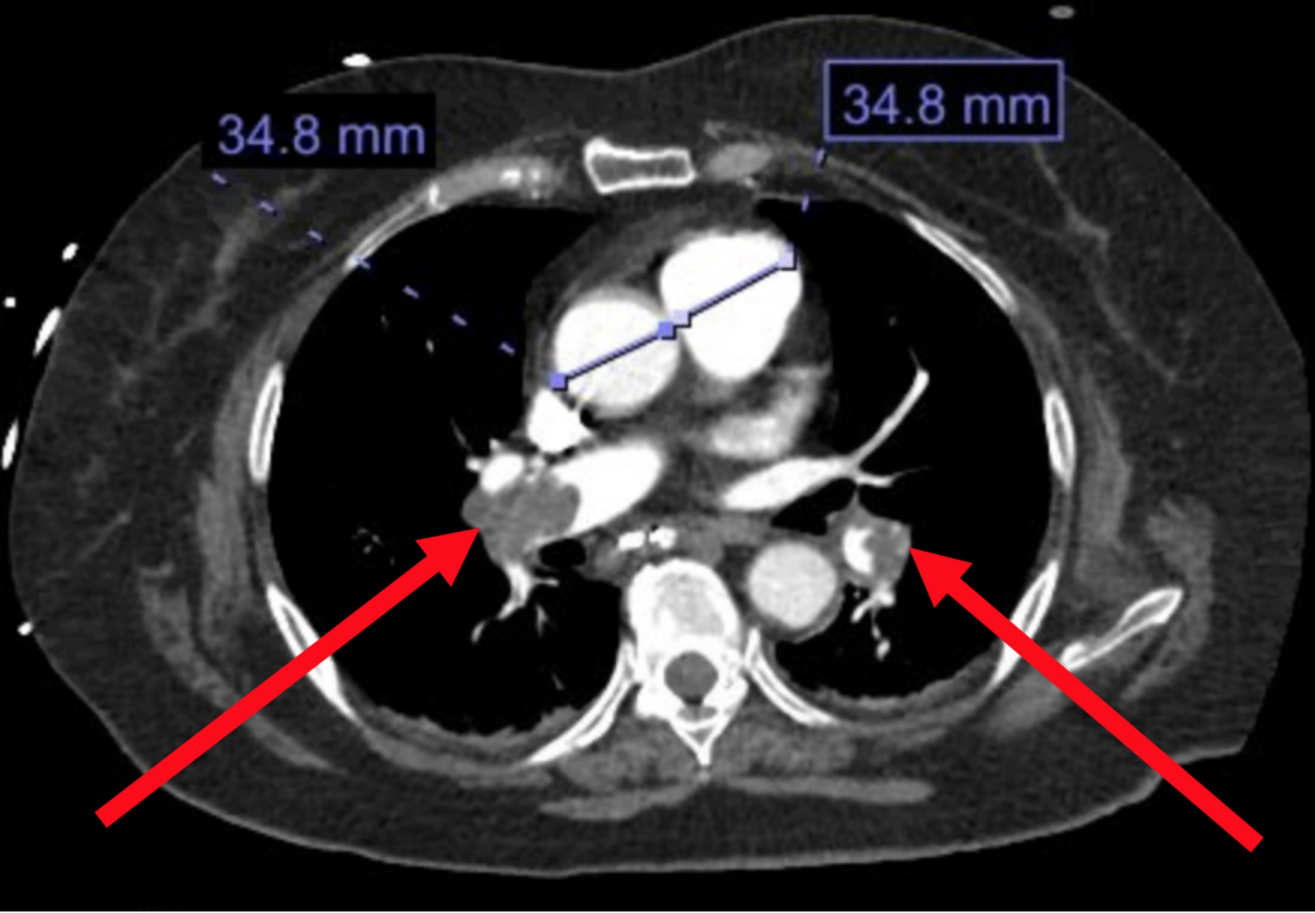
Axial image of CT of chest with intravenous contrast showing right main and left segmental pulmonary artery embolisms (red arrows). Measurements of the diameter of the pulmonary trunk and aorta are both 34.8 mm supporting radiographic evidence of massive pulmonary embolism.
